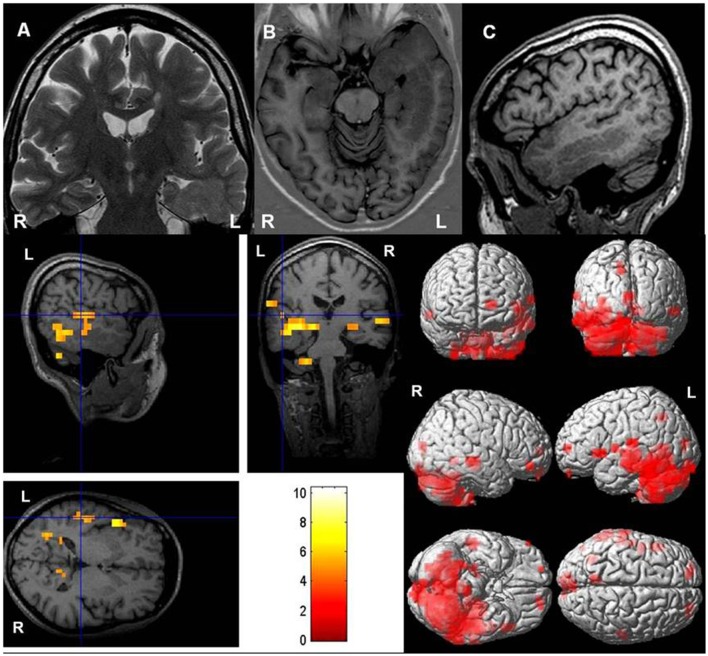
Typical language dominance in a patient with periventricular heterotopia. Upper row: (A) Coronal T2-weighted, (B) axial T1-weighted inversion recovery, and (C) sagittal T1-weighted images show left-sided periventricular heterotopia extending toward cortex. Hippocampus on the left (ipsilateral to periventricular nodular heterotopia) is malrotated. Lower row: Left-hemispheric language dominance with a lateralization index of +75. Blood oxygen level dependent (BOLD) responses are mainly in left Wernicke's and Broca's areas [threshold *p* < 0.05, family-wise error (FWE) corrected].
